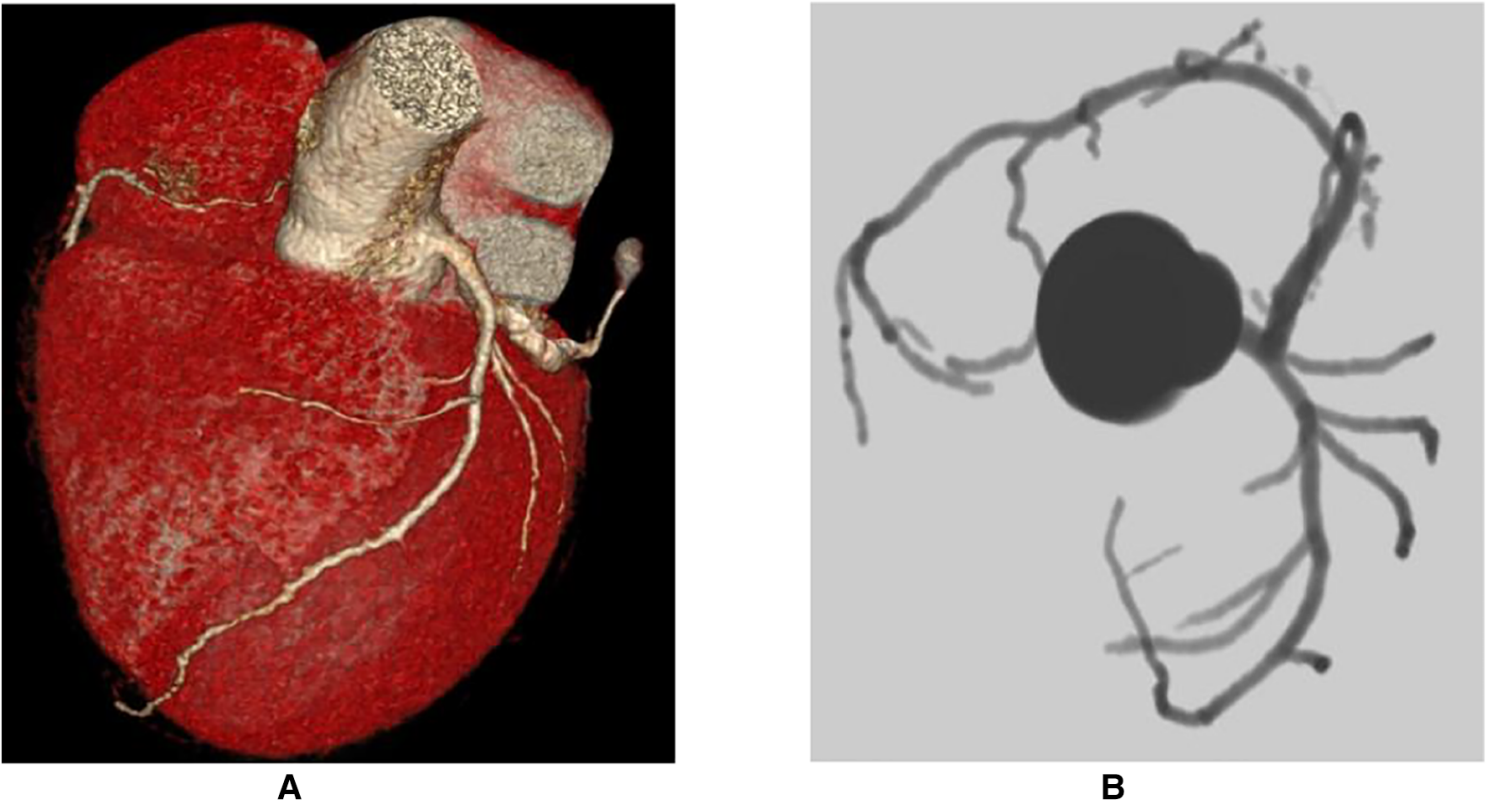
Computed tomography coronary angiogram. (A) Course of the LCx on the posterior atrioventricular groove and continuation of its course in the RCA territory along with take-off of the posterior descending artery. (B) Absence of take-off of the RCA from the right coronary sinus of Valsalva (yellow arrow) and normal origin of the LMS which bifurcates into the LAD and LCX. LAD, left anterior descending artery; LCX, left circumflex artery; LMS, left main stem artery; RCA, right coronary artery.
